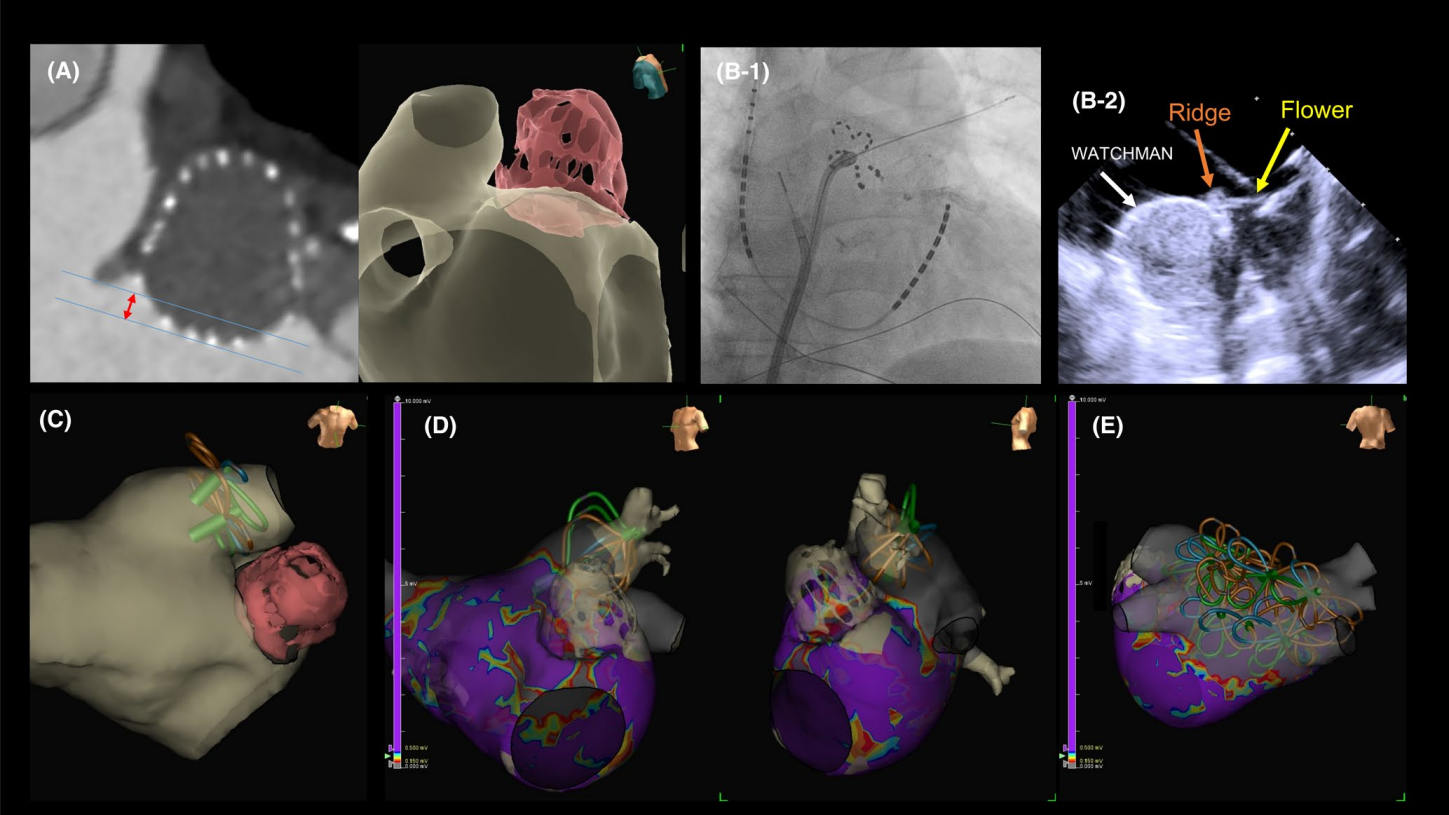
(A) Computed tomography image between LSPV ridge and WATCHMAN (4.4 mm). (B) Image showing no overlap between WATCHMAN and FARAPULSE (B‐1: Fluoroscopic image; B‐2: ICE). (C) Flower configuration application shadows during LSPV isolation. (D) Basket configuration application shadows during LSPV isolation and post‐PFA map. (E) Final post‐PFA map.
